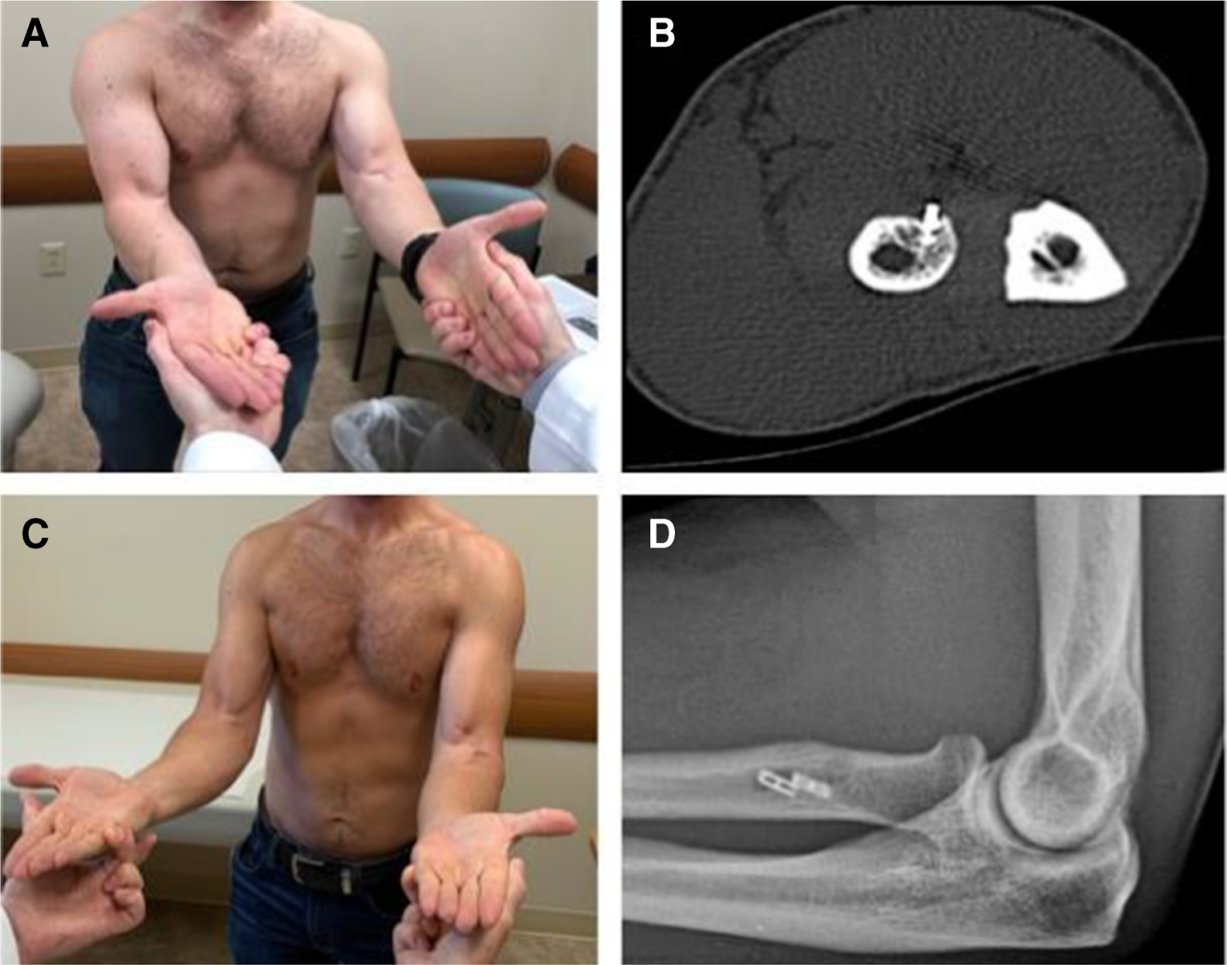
A Photograph of the patient that shows weakness in terminal supination, after distal biceps repair through an anterior approach. This prevents him from competing as a marksman as he cannot stabilize his rifle. B Axial CT scan showing distal biceps anchors placed anterior to the proutuberance, decreasing the moment arm of the tendon, and causing his weakness. C Post-operative photograph showing the improvement in the supination strength after revision repair in an anatomic position though a posterior approach. D Post-operative x-ray showing the anatomic repair of both distal biceps tendon heads with 2 intramedullary unicortical buttons
